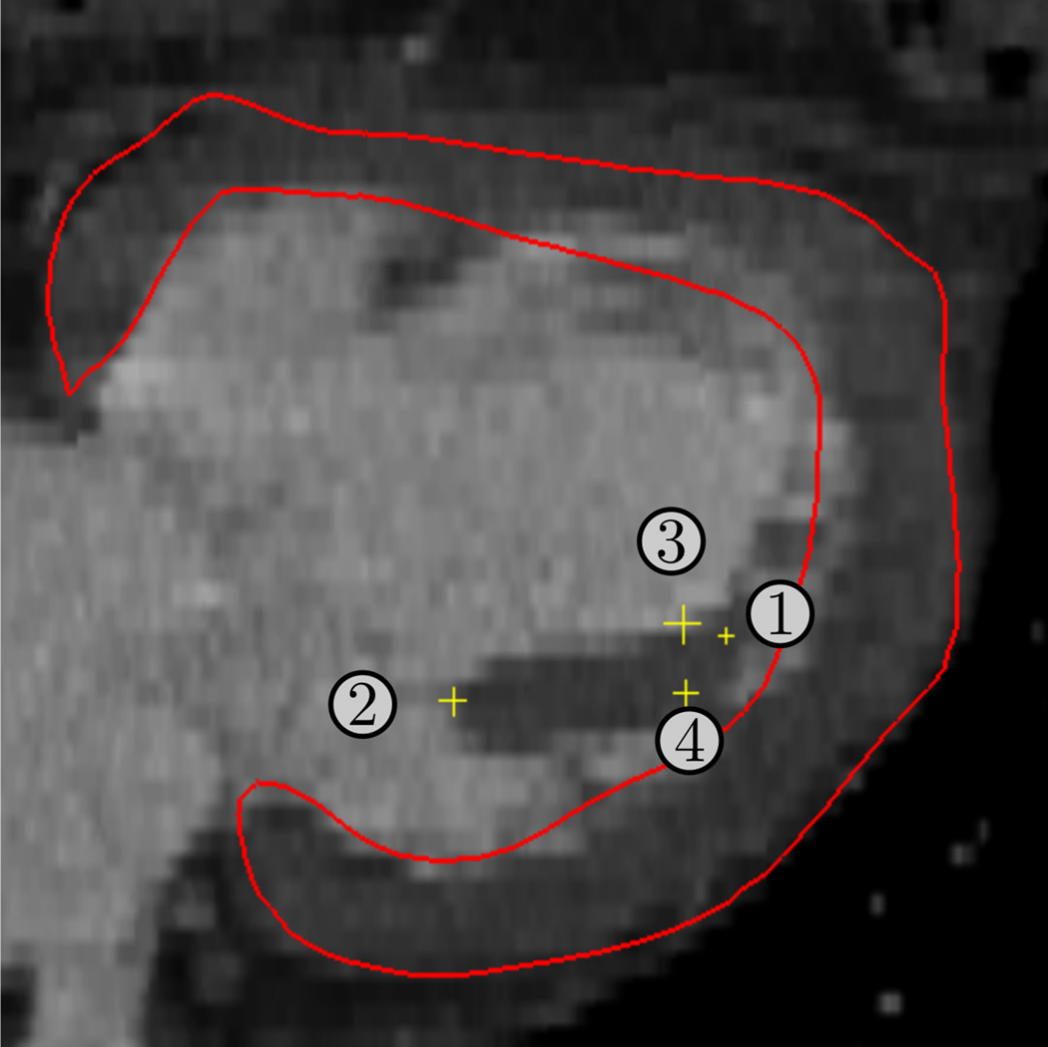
A computed tomography slice and mesh of the left ventricle (red) with the landmarks (yellow). This has been used to build the statistic of the papillary muscle: (1) Attachment point (2) Tip of papillary muscle, (3–4) Landmarks for the diameter estimation.
